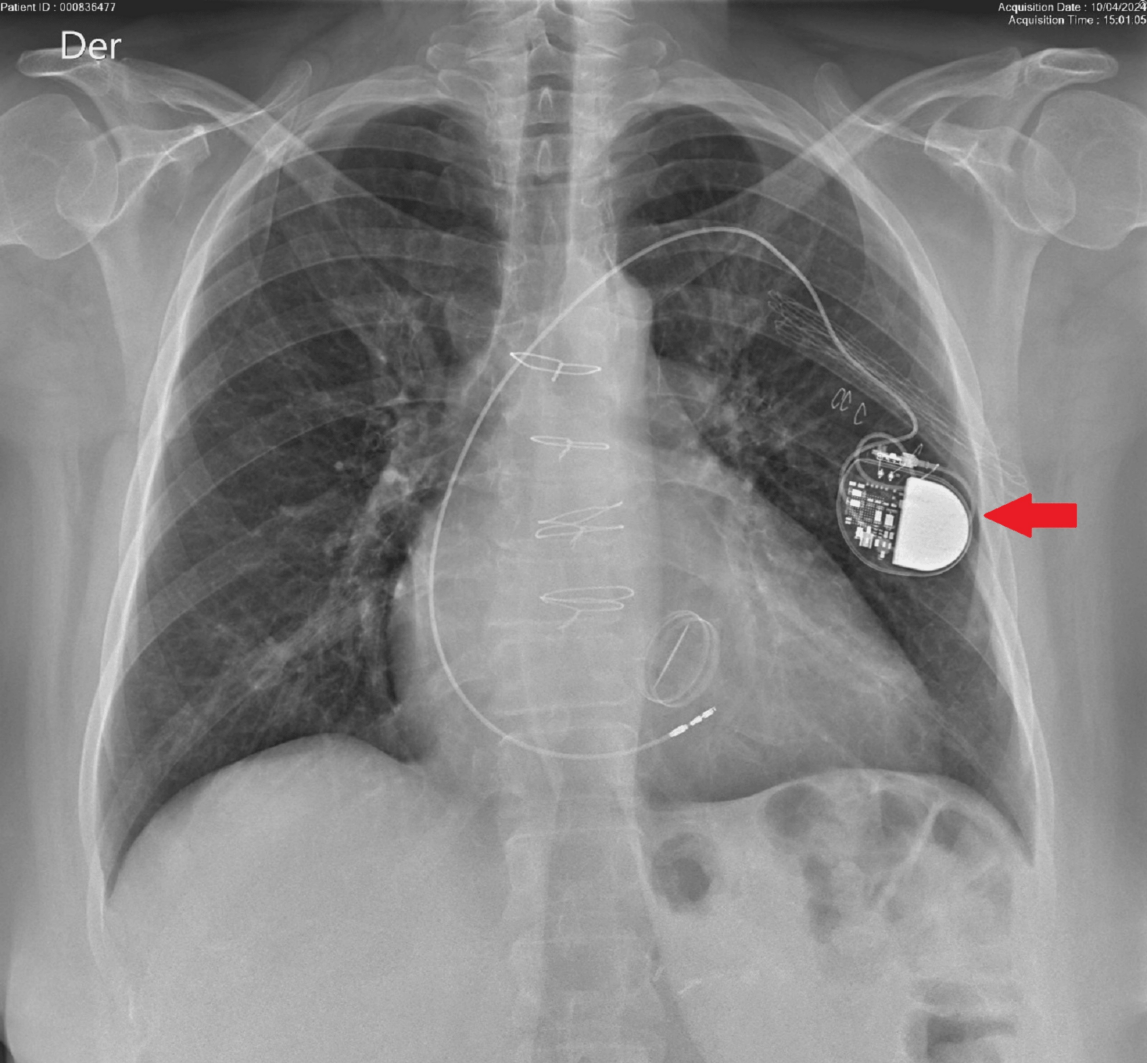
Chest X-ray after pacemaker and ventricular lead repositioning
